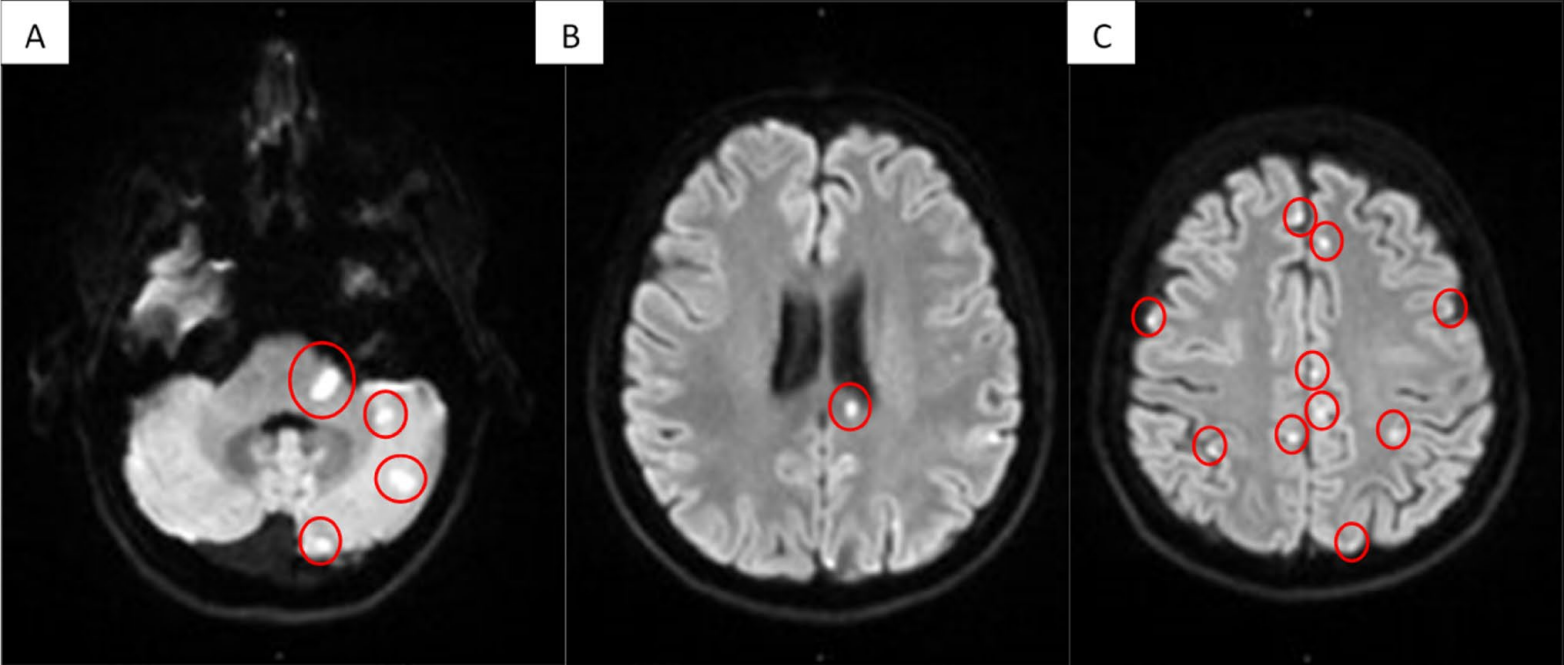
Typical findings of Susac syndrome. Diffusion weighed imaging (DWI) lesions of the left middle cerebellar peduncle and cerebellar hemisphere (a), the corpus callosum splenium (b), as well as in multiple cortical and subcortical localisations (c) on MRI
